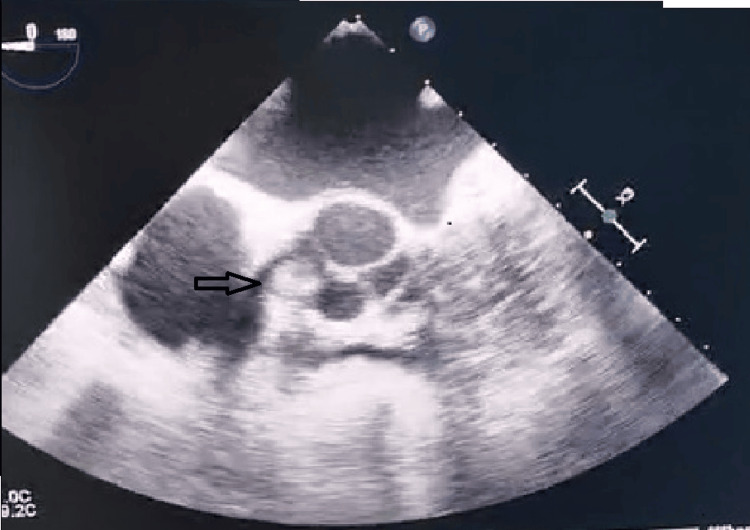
Transesophageal echocardiography (diastolic phase) demonstrating a papillary fibroelastoma arising from the aortic valve.
